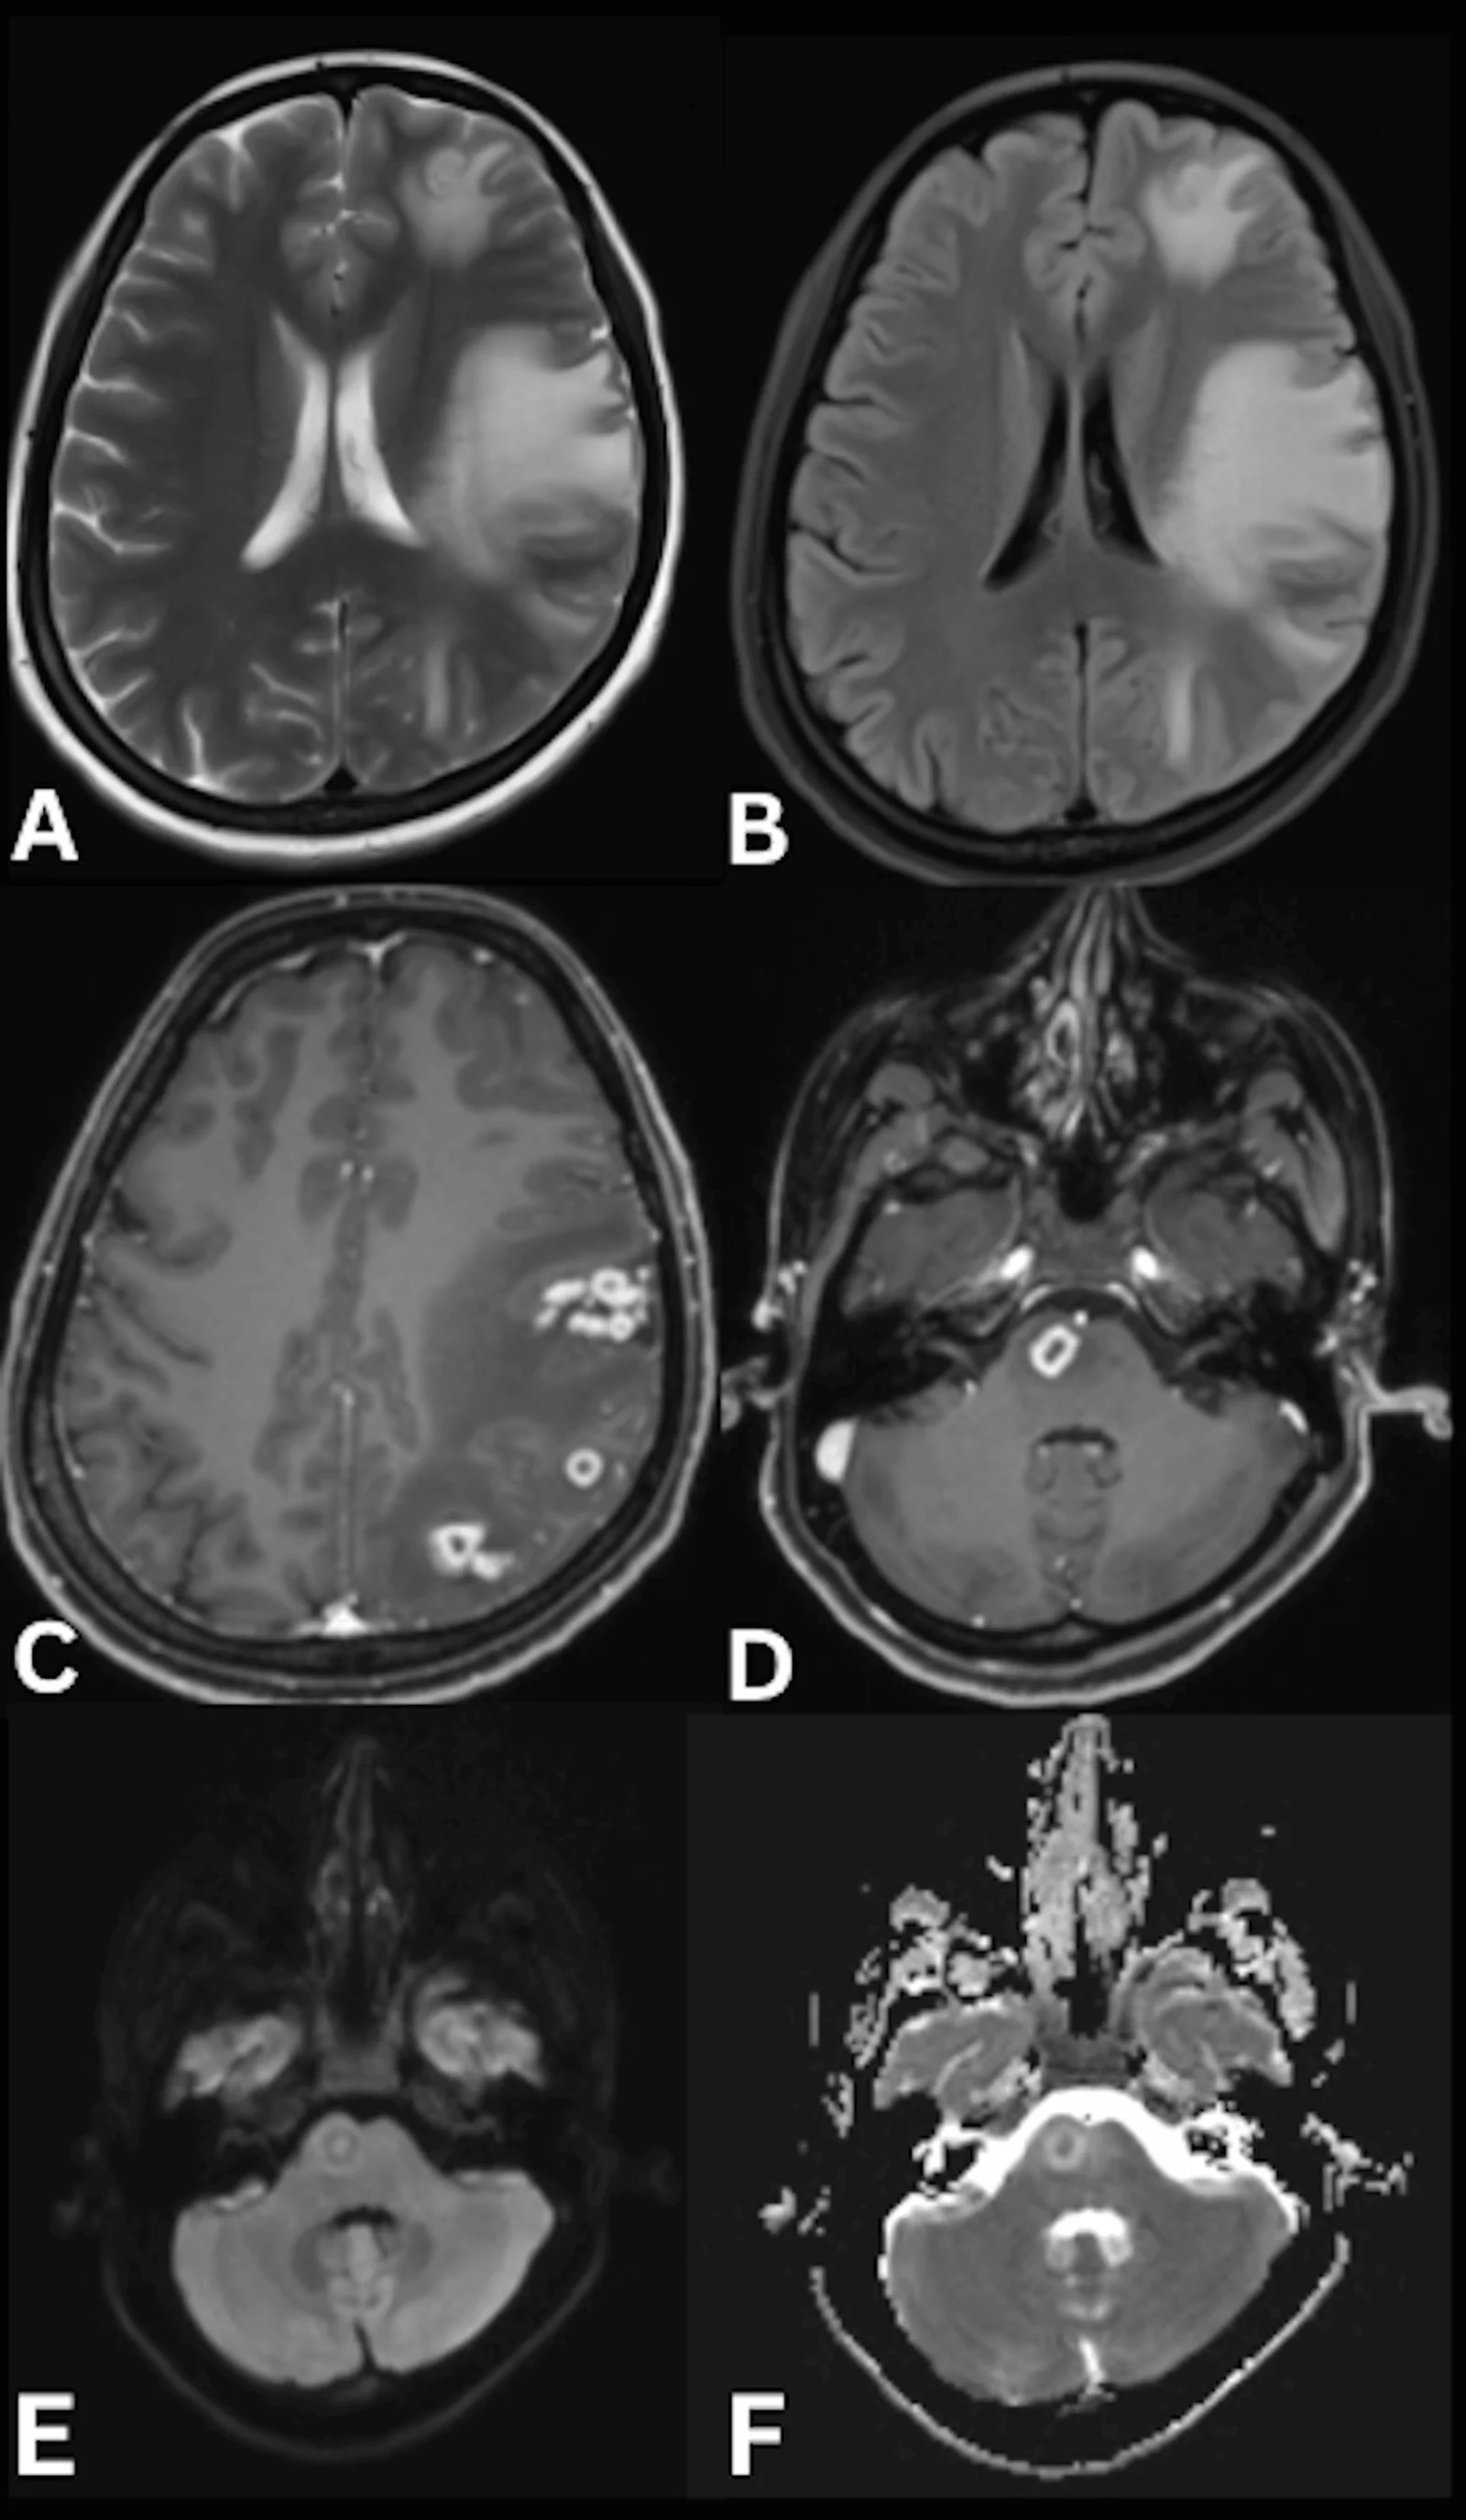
Pre-treatment contrast-enhanced MRI of the brain. (A) Axial T2-weighted, (B) axial FLAIR, (C) and (D) axial post-contrast T1-weighted, and (E) and (F) diffusion-weighted imaging (DWI) and apparent diffusion coefficient (ADC) sequences demonstrate multiple clustered ring-enhancing lesions in the left frontoparietal region, predominantly involving the subcortical white matter, along with a separate enhancing lesion in the right pons. Smaller lesions demonstrate nodular enhancement, whereas the larger lesions demonstrate central diffusion restriction. Moderate surrounding vasogenic edema on the FLAIR images. FLAIR: fluid-attenuated inversion recovery.
